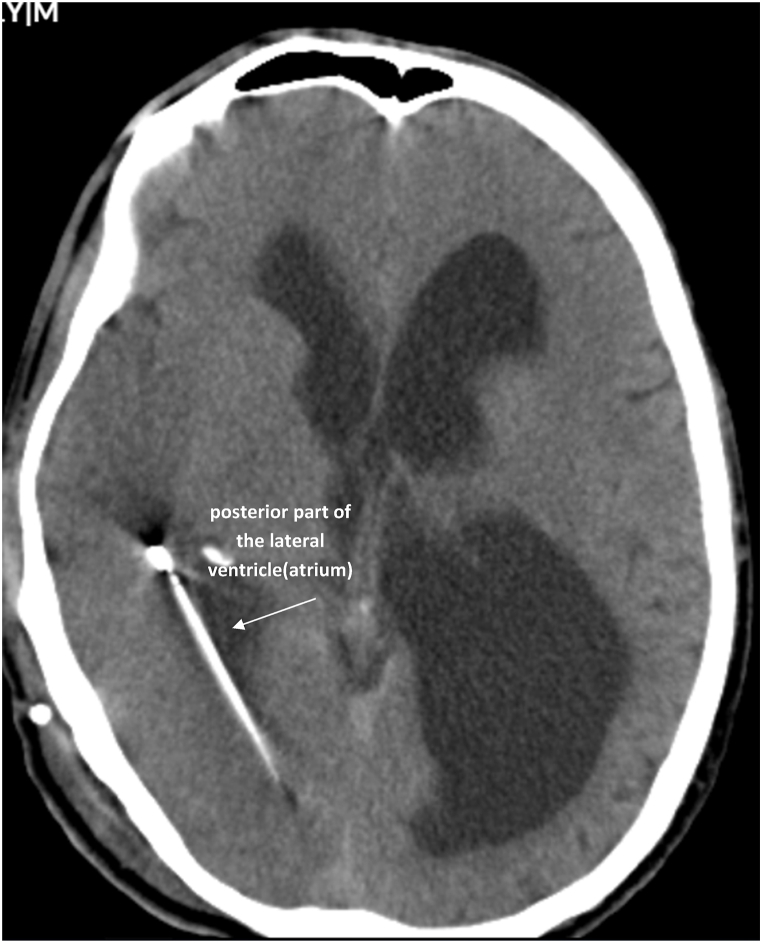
The axial view of the CT scan shows the placement of the ventricular catheter inside the atrium of the right ventricle.
